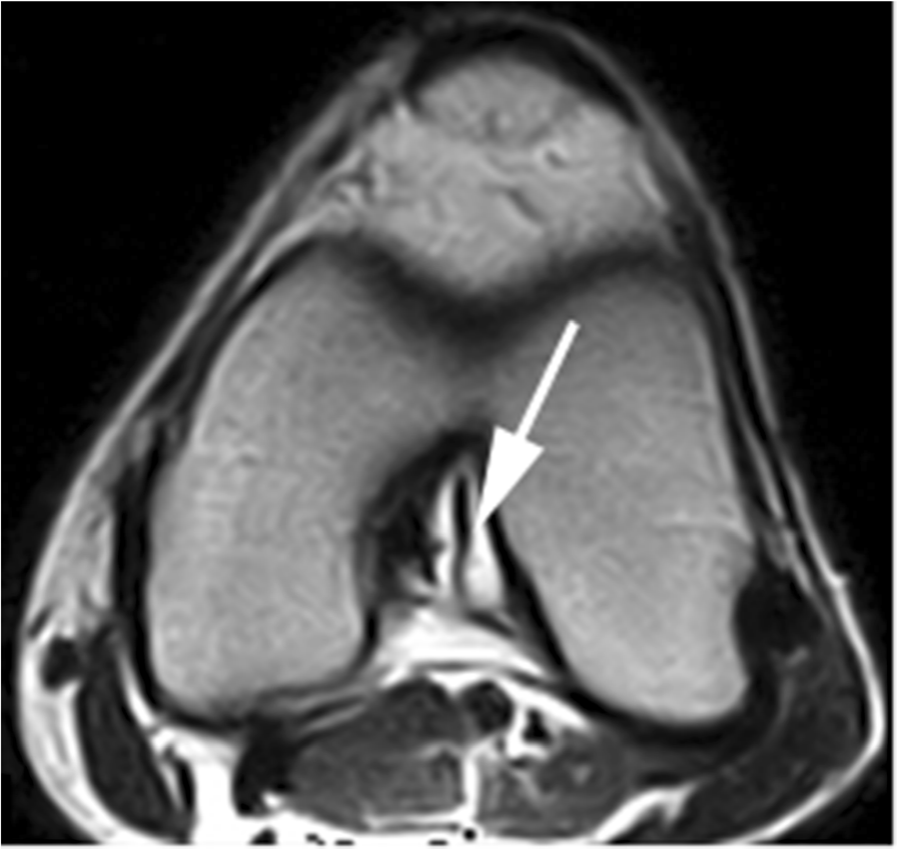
The cyst encompassing the ACL spread between the ACL and PCL on T2-weighted images.
